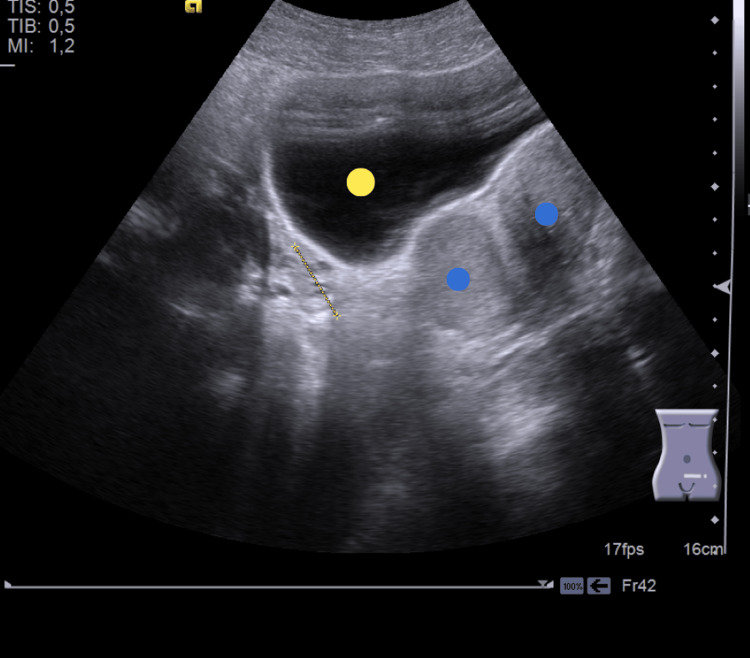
Pelvic ultrasound Blue dots: two uterine cavities. Yellow dot: urinary bladder. Yellow dashes on the left: one ovary TIS: thermal index for soft tissue; TIB: thermal index for bone; MI: mechanical index
